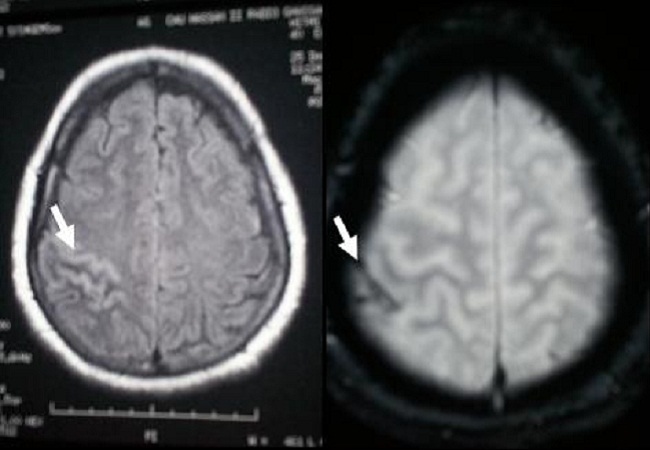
(case 20): FLAIR MR cerebral axial sequences (on the left) showing a hypersignal at the level of the ascending frontal gyrus (arrow) and in T2* (on the right) showing a hyposignal of a cortical vein (arrow) which is thrombosed
